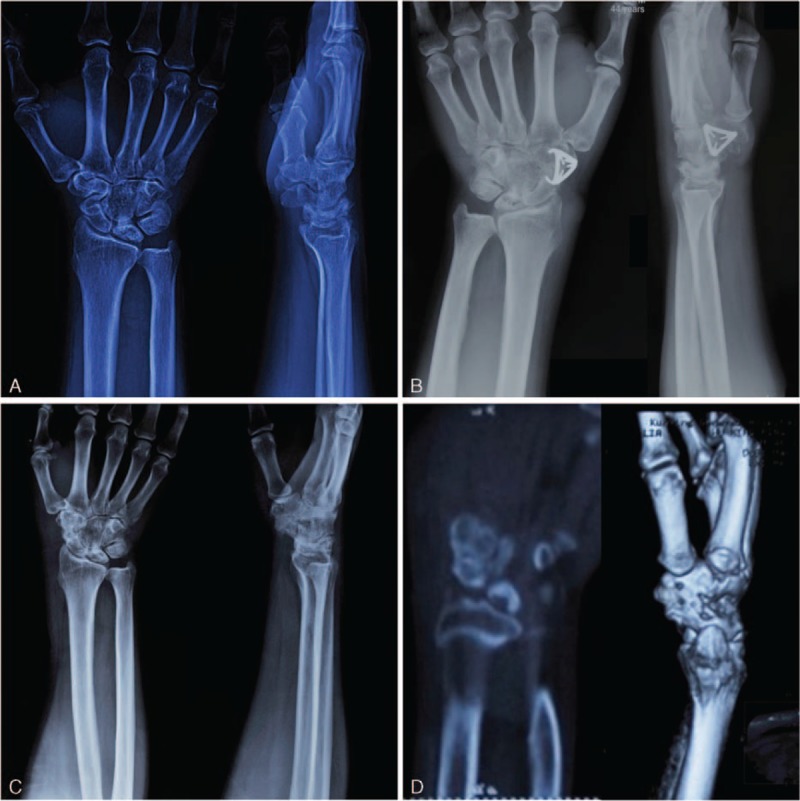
Radiographs of a 44-year-old man. A, Preoperative X-ray images showing rotation and laxation of the lunate bone, and increased scapholunate angle. B, Postoperative X-ray images showing the reduced lunate bone, normal scapholunate angle, and position of the implant. C, X-ray images after implant removal showing fusion of the scaphoid, trapezium, and trapezoid, and no further collapse of the lunate bone. D, Computed tomography images after implant removal showing the fused scaphoid, trapezium, and trapezoid.
